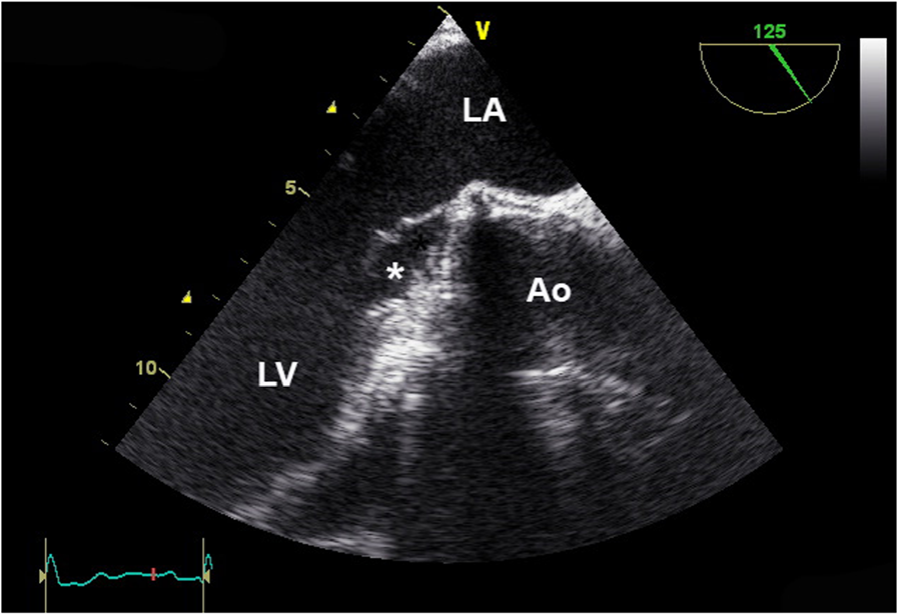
Photograph obtained during transesophageal echocardiography (long axis view of the left ventricular outflow tract). Two vegetations (*) are visible on the mechanical bileaflet aortic valve, protruding into the left ventricular outflow tract in diastole. Ao = aorta, LA = left atrium, LV = left ventricle
